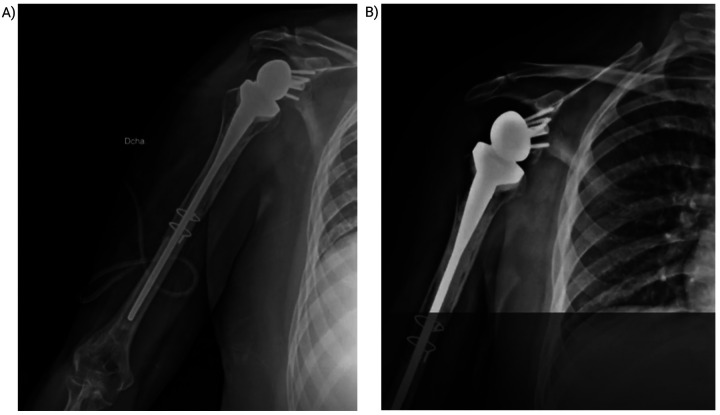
Postoperative control and follow-up. (A) Immediate postoperative radiograph showing reverse shoulder prosthesis with cerclage wire and “flute-beak” osteotomies. (B) Radiograph at 5 months postoperative demonstrating early osteointegration at the graft-host interface.
